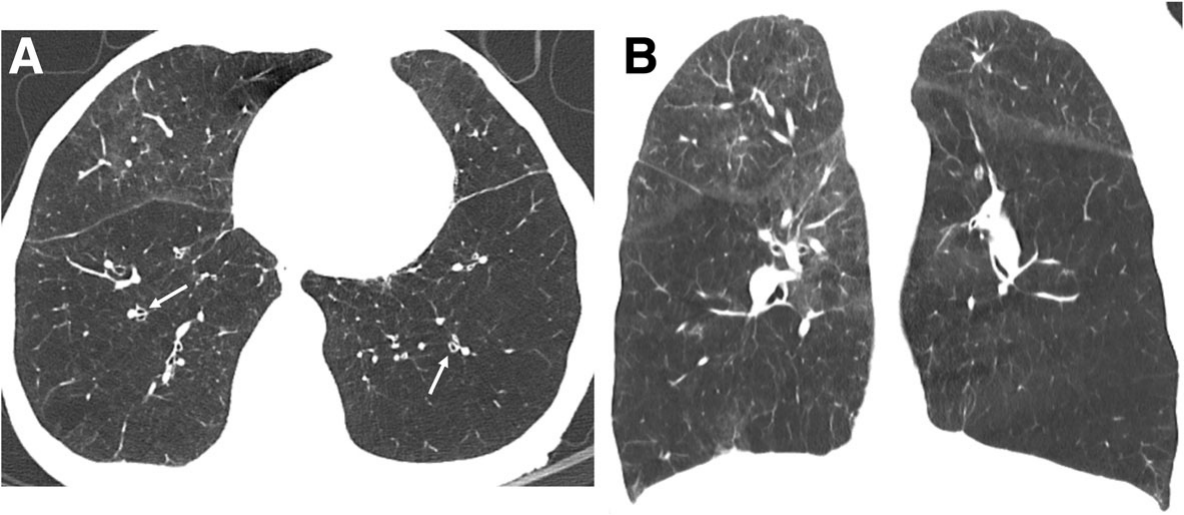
PLE in a 51-year-old man with alpha-1 antitrypsin deficiency. a Axial and b coronal CT images show hyperinflation and lower lobe predominant emphysema, involving the entire secondary pulmonary lobules. Bronquial wall thickening is also depicted (arrows)
